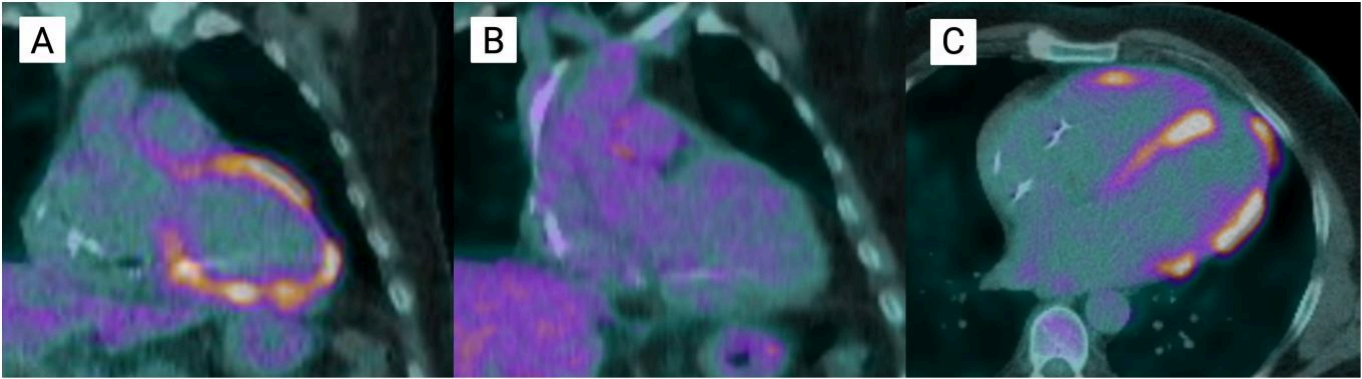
Fluorodeoxyglucose changes in response to immunosuppression. (*A*) In May 2024, fluorodeoxyglucose positron emission tomography computed tomography showed increased metabolic activity in nearly the entire left ventricle and part of the right ventricle, in keeping in active sarcoid related inflammation. (*B*) In November 2024, following treatment with high-dose intravenous glucocorticoids, repeat imaging showed complete response to treatment without increased fluorodeoxyglucose activity in the heart. (*C*) There is new low-grade increased fluorodeoxyglucose uptake in the mid-inferolateral wall and mid-inferoseptal wall, which may represent recurrence of low-grade cardiac inflammation. FDG PET, fluorodeoxyglucose positron emission tomography; CT, computed tomography.
